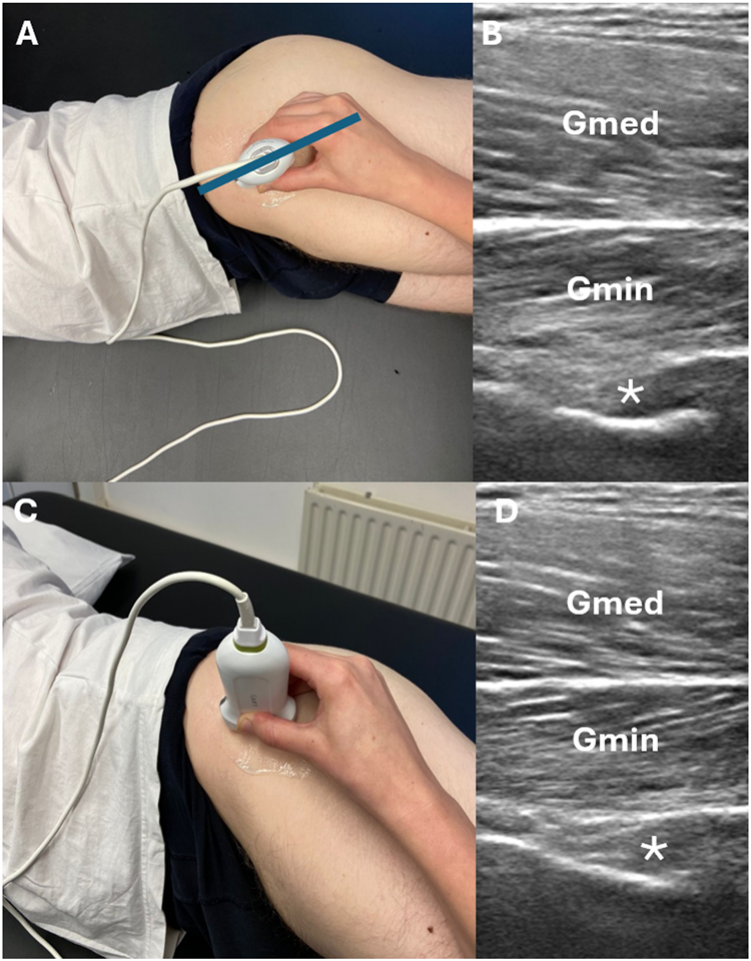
(A) Overview of probe placement, the blue line gives a representation of the angle. (B) Longitudinal ultrasound view of the gluteus medius (Gmed) and gluteus minimus (Gmin), Asterisk * shows the bony landmark. (C) Overview of the probe placement from a frontal‐sagittal view. (D) Shows the longitudinal ultrasound view of the Gmed and Gmin.
